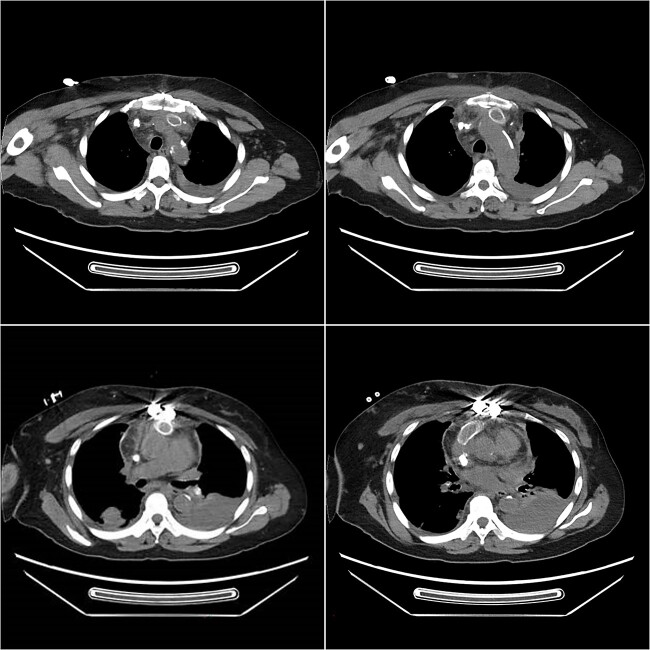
Postoperative computed tomography angiography (CTA) showed no contrast agent leakage in the artificial blood vessel.
